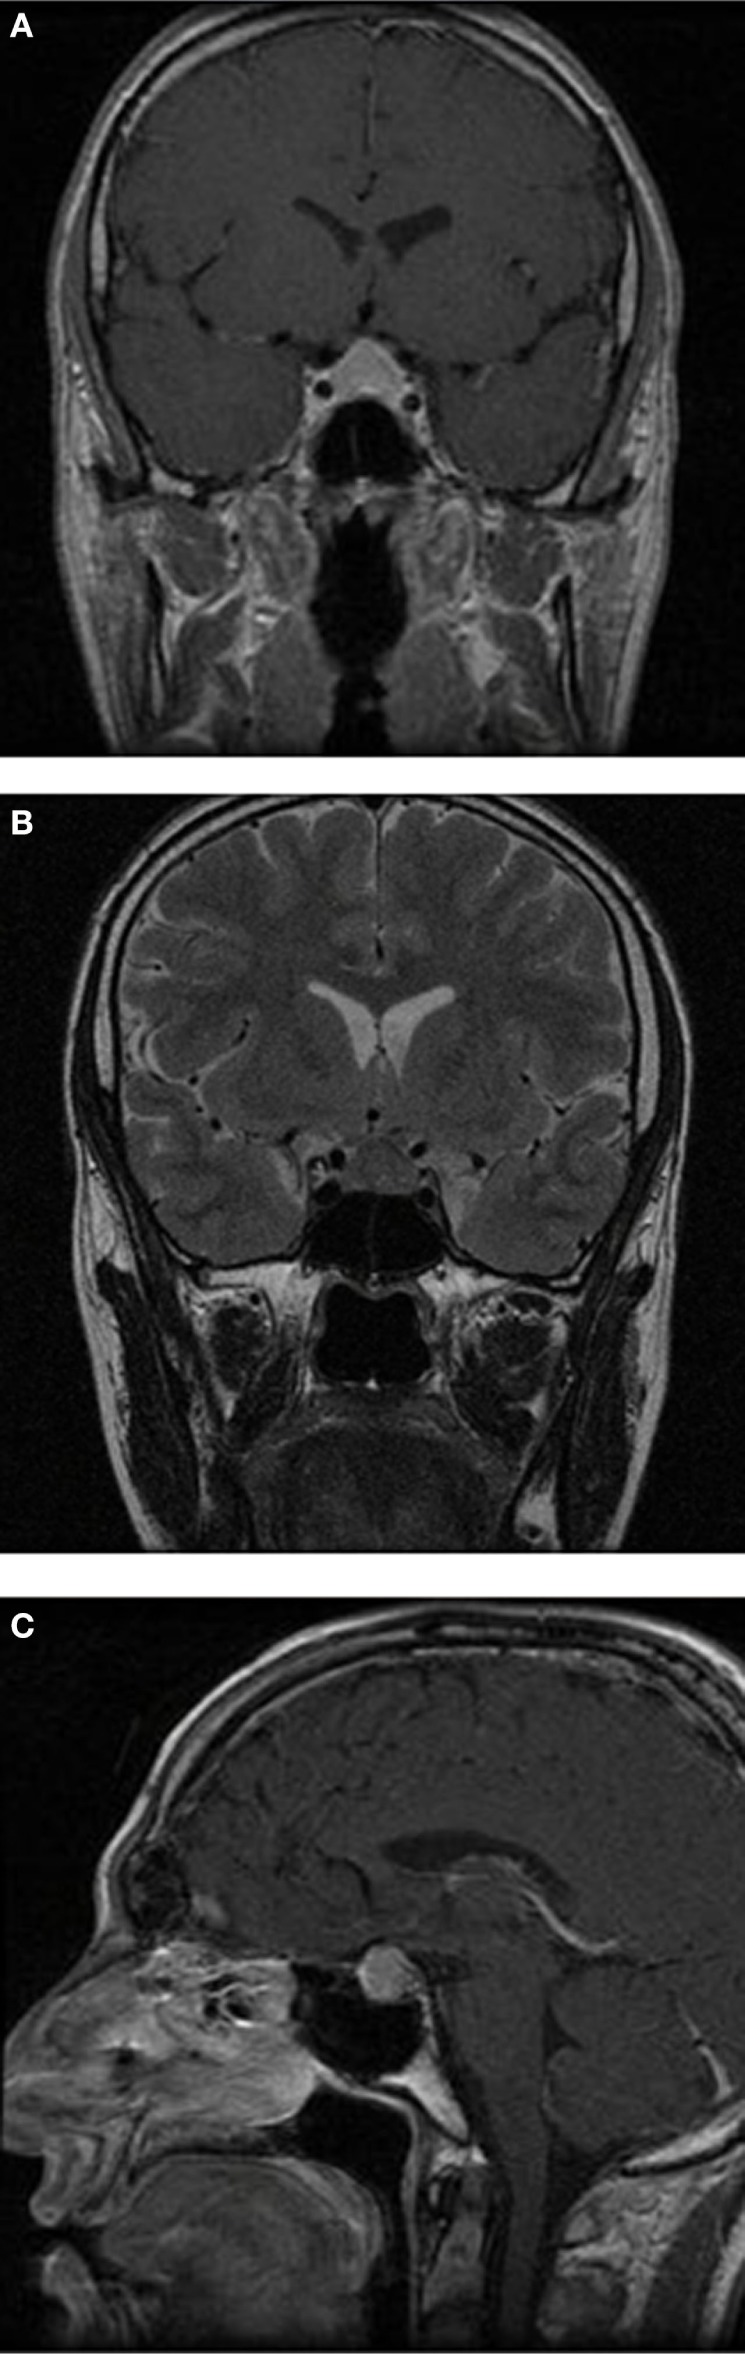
Brain magnetic resonance imaging (MRI) sections. Brain MRI, showing a homogenous and diffuse pituitary enlargement of up to 1.1 cm, which extended to the suprasellar region and contacted the optic chiasm, blurring of the pituitary stalk, absence of clear differentiation between the anterior and posterior lobes, and no signs of hemorrhage or adenomas. (A) Frontal T1-weighted after gadolinium; (B) frontal T2-weighted; and (C) sagittal T1-weighted after gadolinium.
